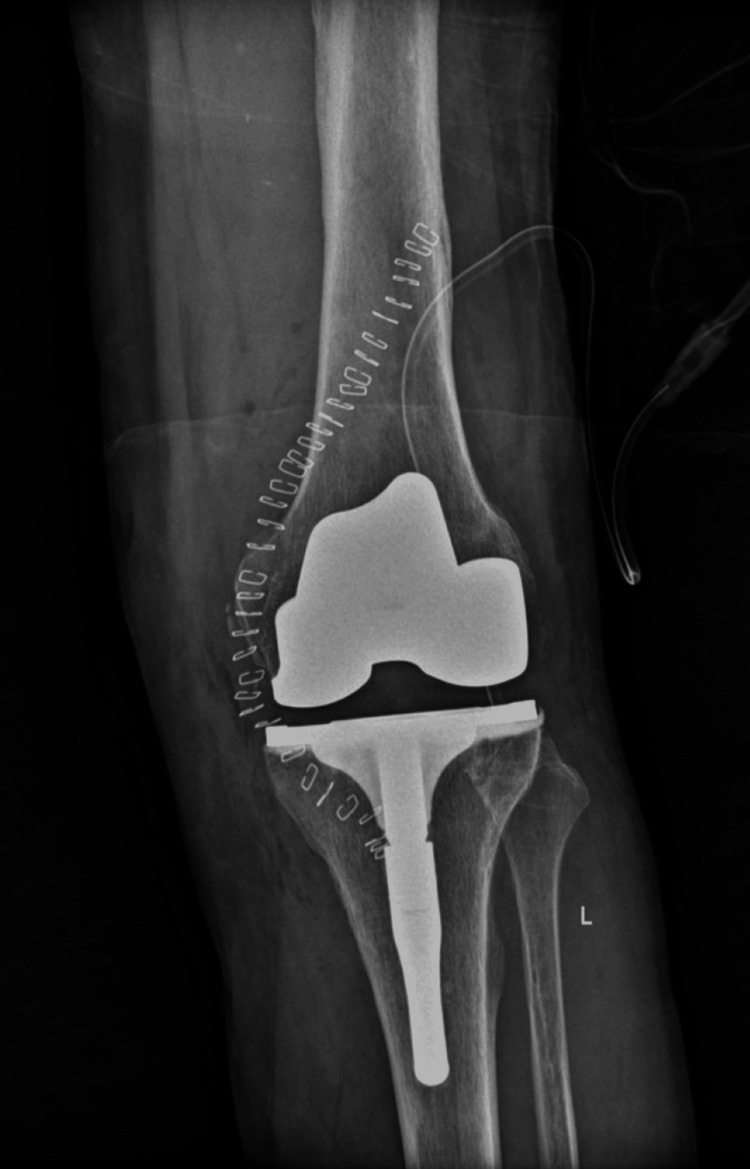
Postoperative Anteroposterior X-ray of the Left Knee
